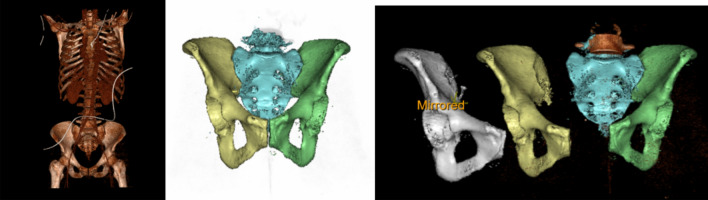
3D CT module showing the steps in the reconstruction.
